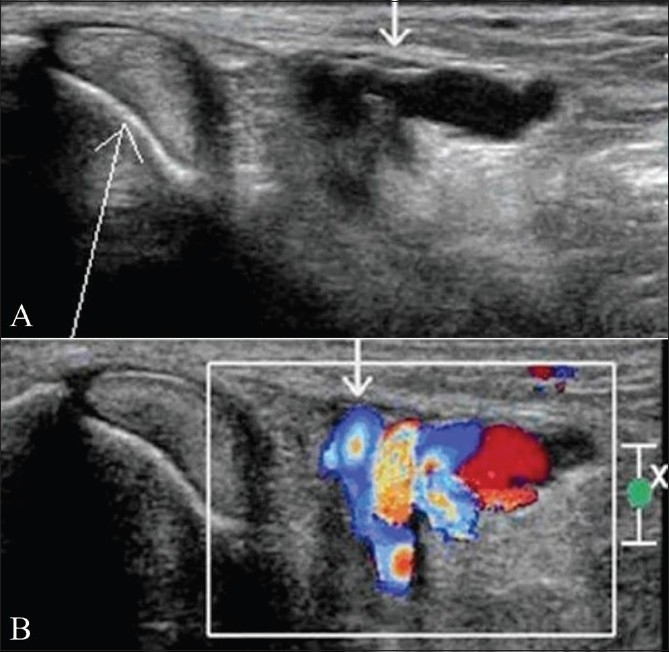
Vascular malformation in the tarsal tunnel causing compressive posterior tibial neuropathy. Transverse gray-scale USG (A) shows dilated and tortuous vessels (small arrow) with flow on color Doppler (B) in the right tarsal tunnel adjacent to the tibialis posterior tendon (long arrow)
